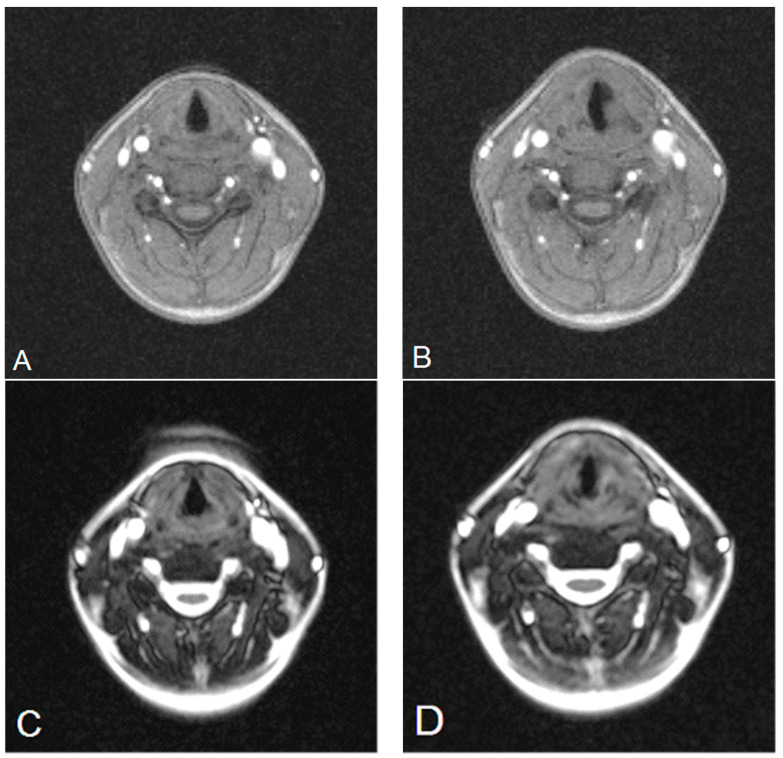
Images of left vocal fold paralysis (B,D) compared to preoperative examination (A,C). MRI-GRE (A,B), MRI-TRUFI (C,D).
